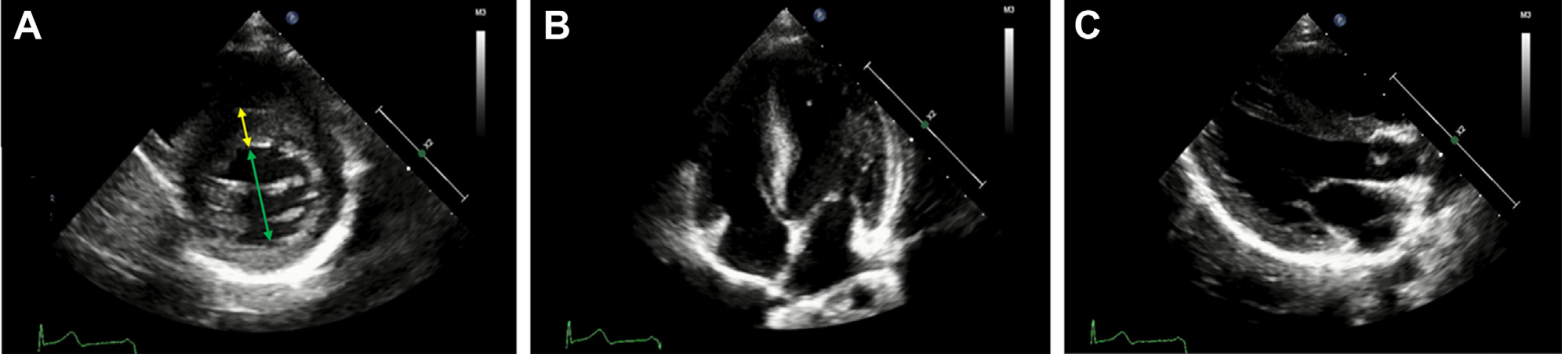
Echocardiography performed on the 14th day of admission, which revealed similar findings to that performed on the day of admission; maximum intraventricular septum, 13 mm (yellow double-sided arrow) and left ventricular end-diastolic diameter, 33 mm (green double-sided arrow). There was no evidence of left ventricle outflow tract stenosis; the peak velocity was 1.5 m/s. No signs of systolic anterior movements of the anterior mitral leaflet were observed. (A) Parasternal short-axis view at end-diastole; (B) apical 4-chamber view at end-diastole; (C) parasternal long-axis view at end-diastole.
